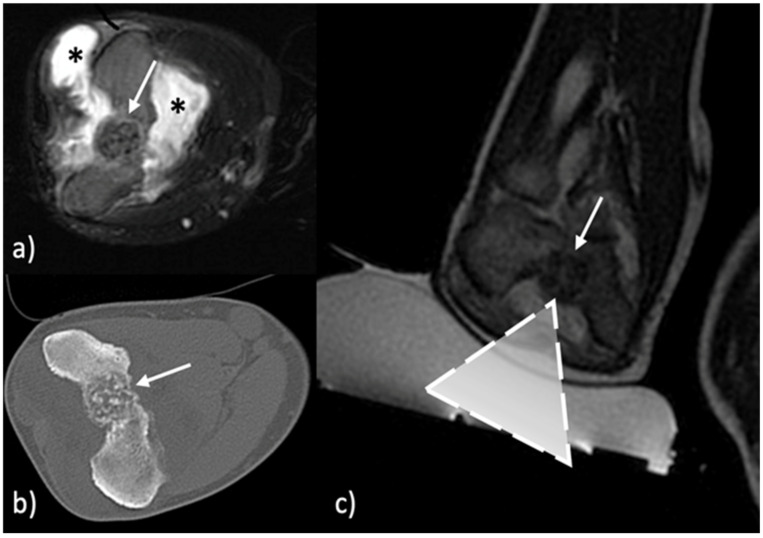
Intra-articular osteoblastoma of the elbow (a) Pretreatment T2-weighted fast spin-echo MR with fat-saturation shows the lesion (arrow), bone edema and the reactive intra-articular fluid (asterisk). (b) Pretreatment CT shows the nidus (arrow) of the osteoblastoma. (c) MR image during treatment with the US transducer in contact with the skin (dashed triangle represents the US beam focused on the target indicated by the arrow).
